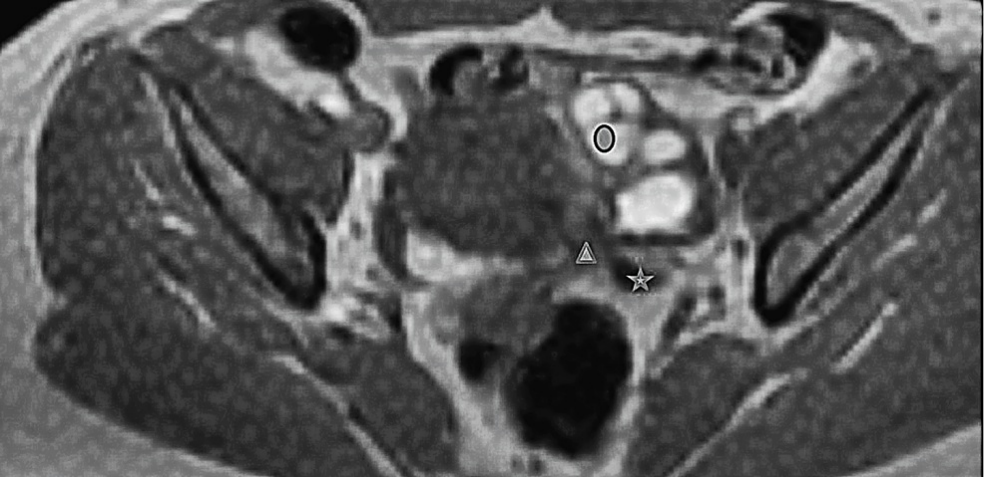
T1 FSE MRI sequence image showing the presence of a left ureteral stenosis (Star), with the presence of a spiculated lesion on the left parametrium (Triangle). A hematosalpinx could also be identified.
